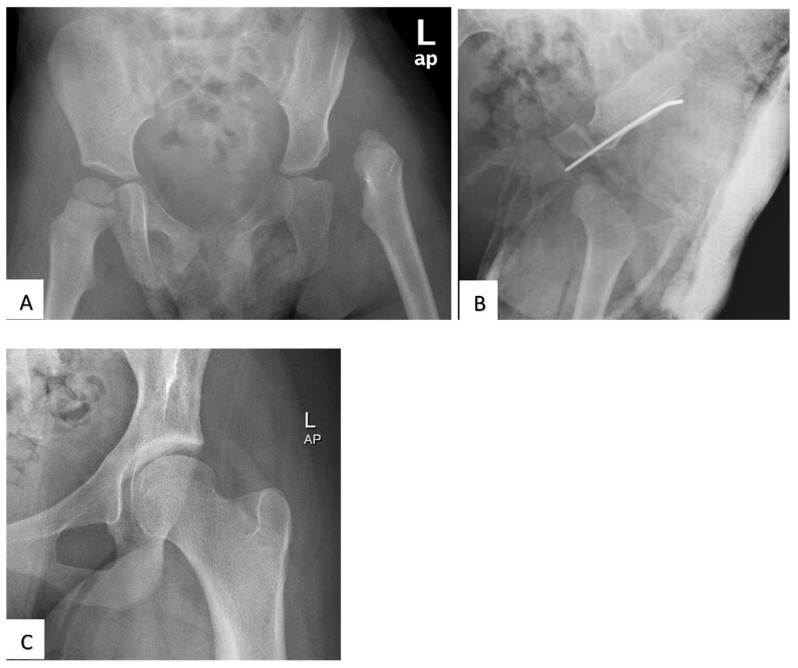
Radiographs of a boy treated with open reduction and Salter osteotomy. He had an excellent clinical outcome (McKay grading). (A) Pre-operative anteroposterior pelvic radiograph at age 20 months showing a left hip dislocation and a dysplastic left acetabulum. (B) Post-operative hip radiograph shows hip reduction and the Salter osteotomy stabilised with a K-wire. (C) Anteroposterior left hip radiograph showing a normal femoral head shape and acetabulum (Severin class I) at age 11 years old.
